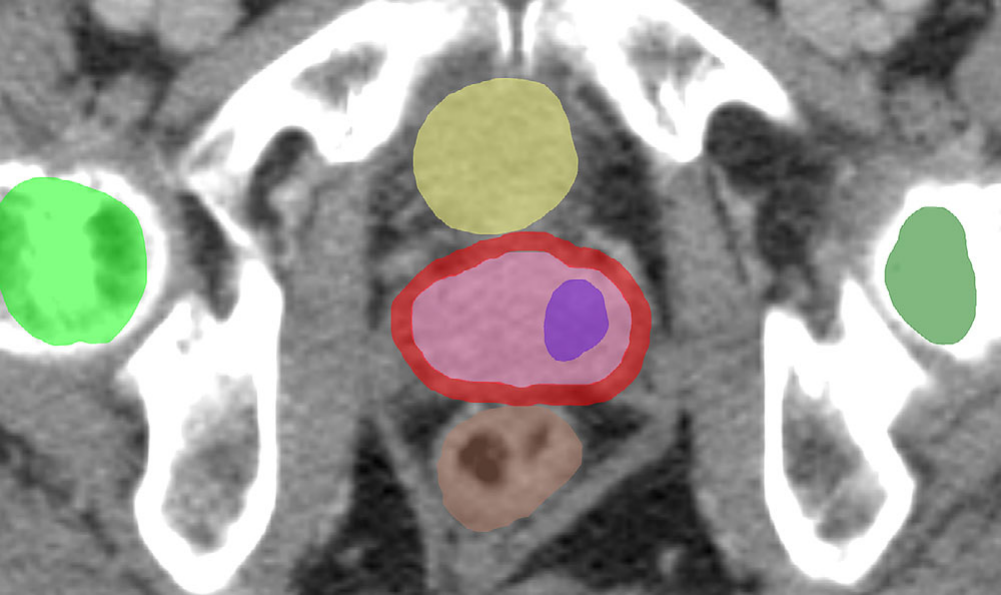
Planning‐CT of an example patient in axial view with TVs and OARs delineated. Visible are GTV_union_ (purple), Prostate^−^ (pink), CTV^−^ (red), Rectum (brown), bladder (yellow), and femoral heads (left: dark green, right: light green).
